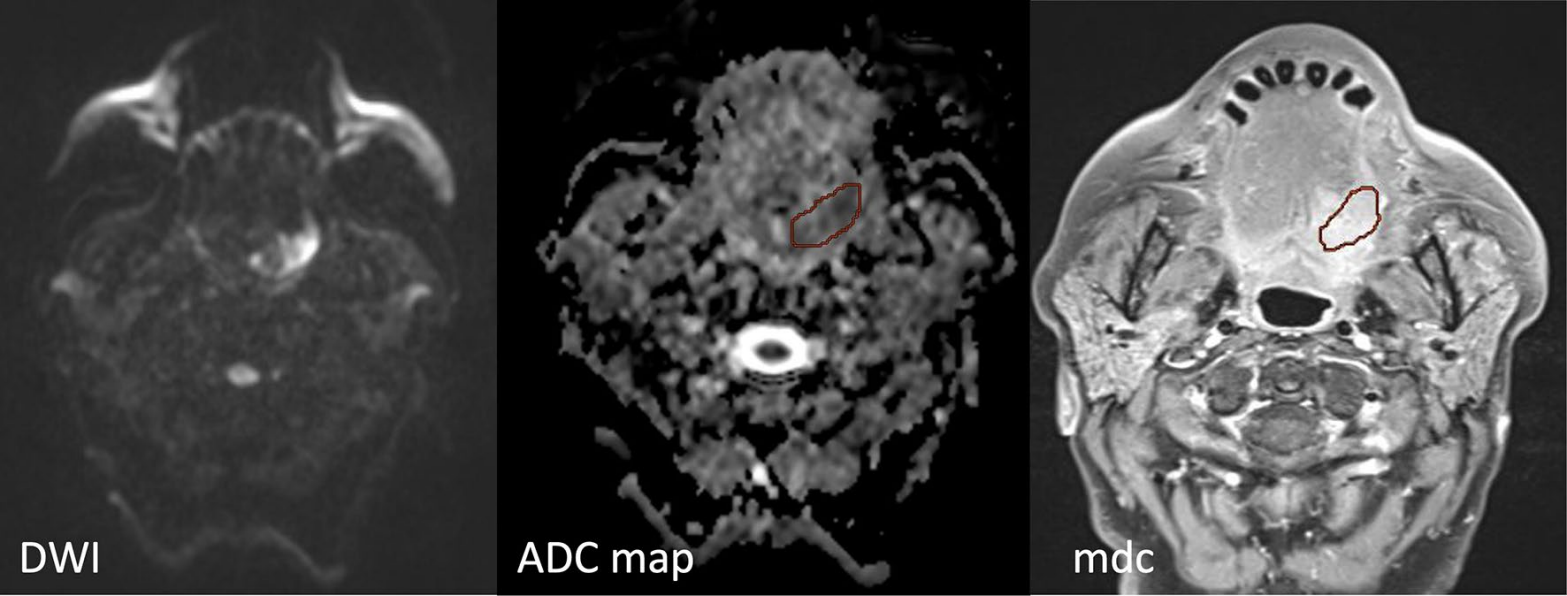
Region of interest (ROI) manually delineated on the contrast-enhanced comparing them with ADC map imaging excluding peritumoral edema. ROIs on the ADC map was based on the lesion observed in the DWI sequence
